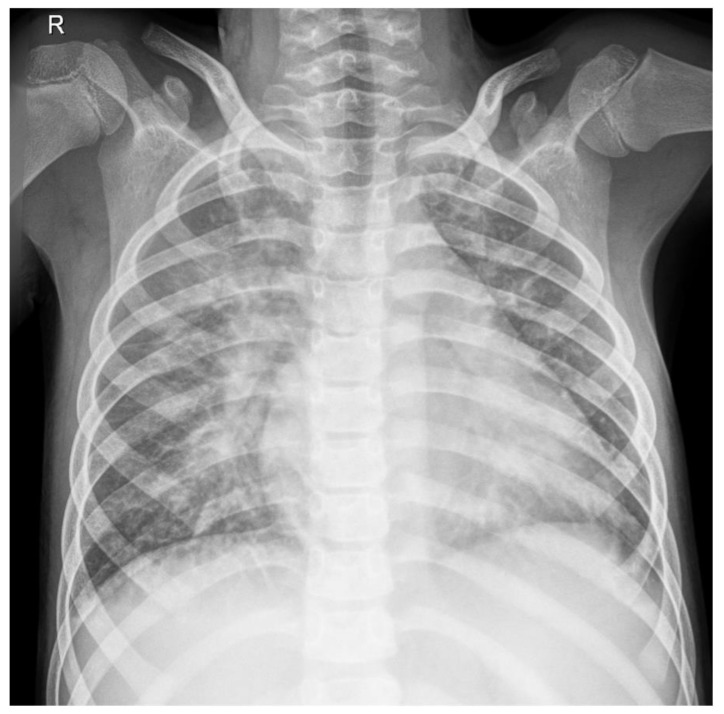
Chest X-ray showing veiling of both costo-diaphragmatic recesses and numerous reticulonodular opacities, with erased contour and tendency to confluence, diffusely distributed in both lung fields. R, right.
